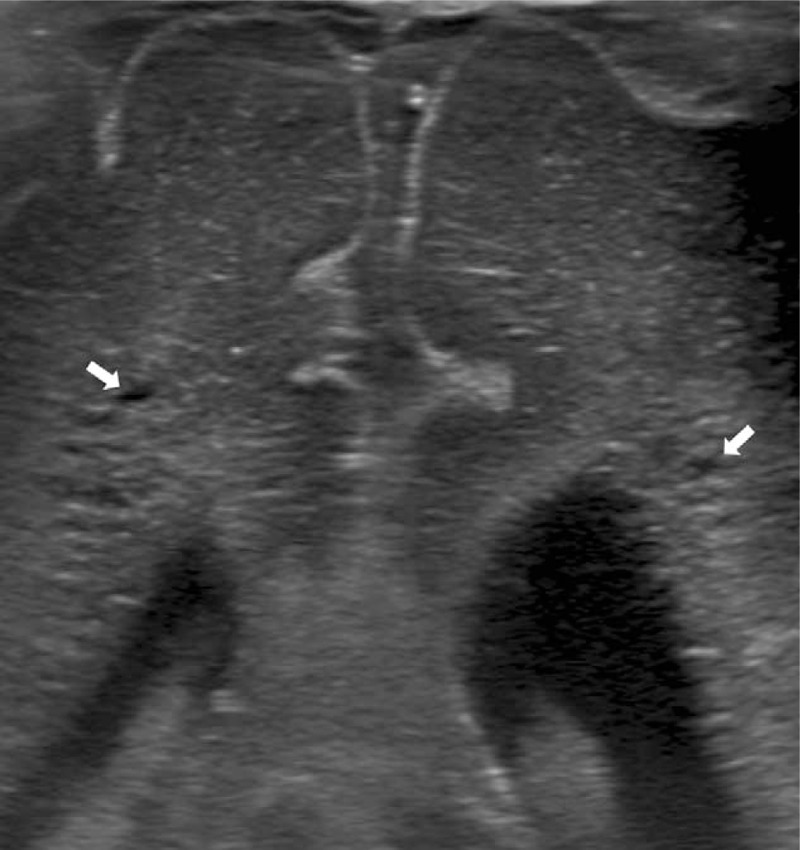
Coronal, magnified view of gray scale cranial sonogram at the level the atria of the lateral ventricles on postnatal day 16 demonstrates white matter damage composed of bilateral increased periventricular echogenecities with small cystic degenerations (white arrows).
